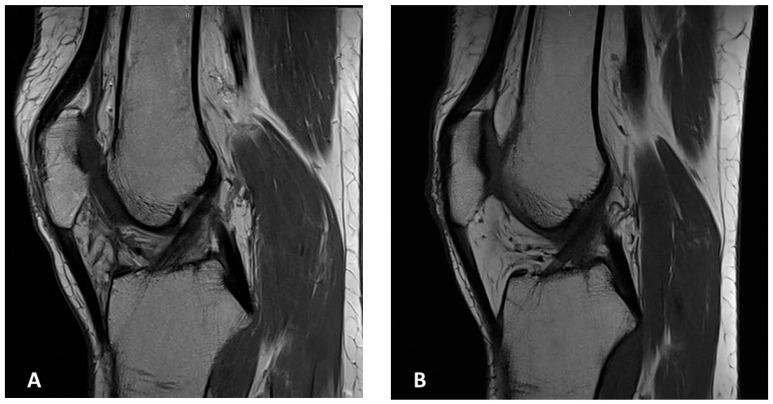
MR images of both knees. Sagittal MR images of the right (A) and left (B) knee at the latest follow-up showing no evidence of local recurrence.
